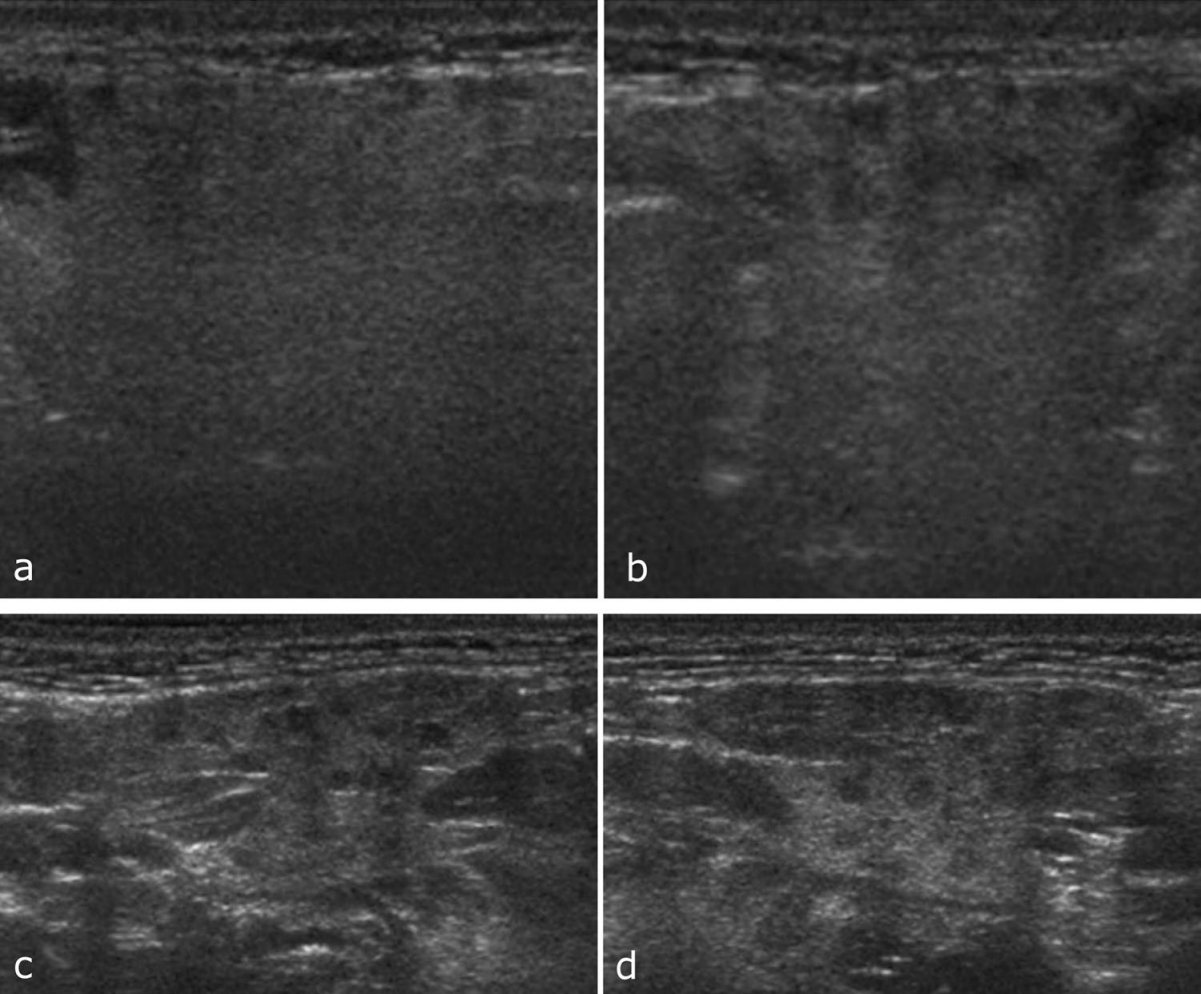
Ultrasonography of the patient shown in Fig. 1 (case 1 in Table 1). a, b, c, d Ultrasound images show multiple oval and polygonal hypoechoic areas in right (a) and left (b) parotid gland parenchyma and in right (c) and left (d) submandibular gland parenchyma
